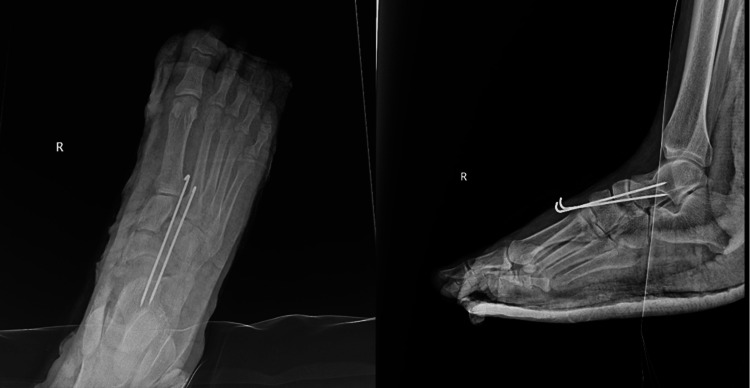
Postoperative X-ray films showing the fixation.
